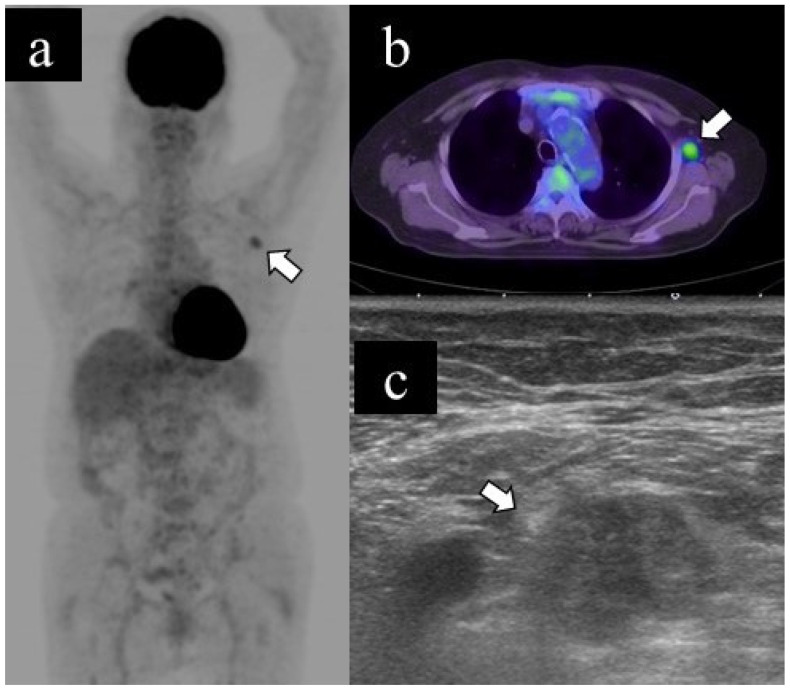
Representative case of malignant lymphadenopathy. (a) Maximum-intensity projection (MIP), (b) transaxial 18F-FDG PET/CT image and (c) US image of malignant lymphadenopathy.
